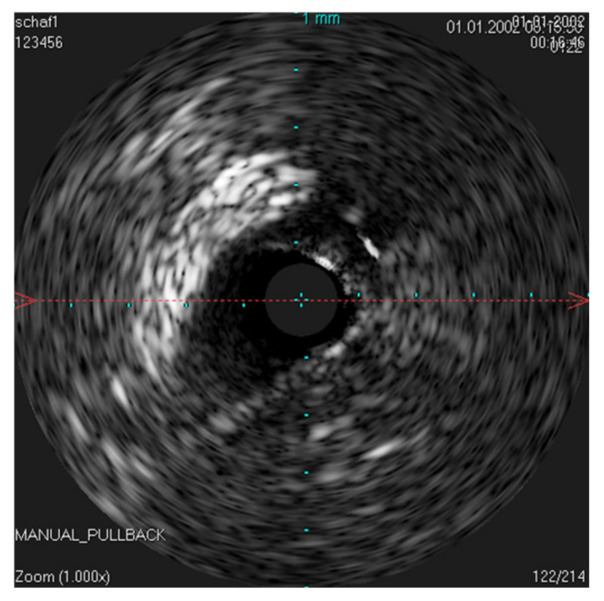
Sonographic image of the ET lumen flushed with water, near to the ET isthmus. The lumen now appears spindle shaped.
